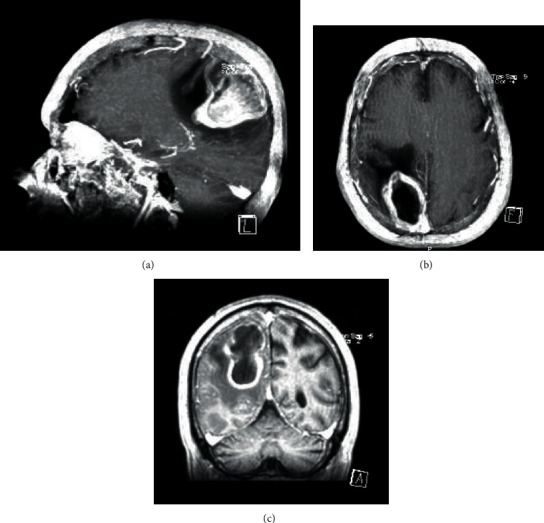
CE-MRI in Hospital São José.
